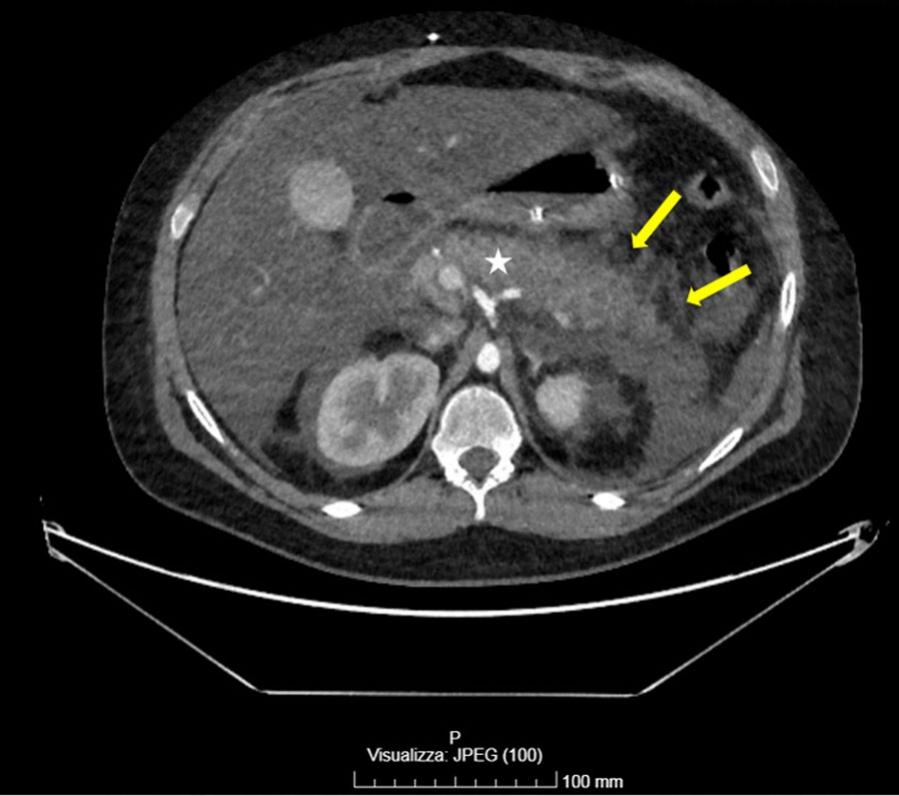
Abdominal contrast-enhanced CT scan, arterial phase, showing a globular pancreas increased in volume (white ✩) along with peripancreatic necrotic areas (yellow arrows)
